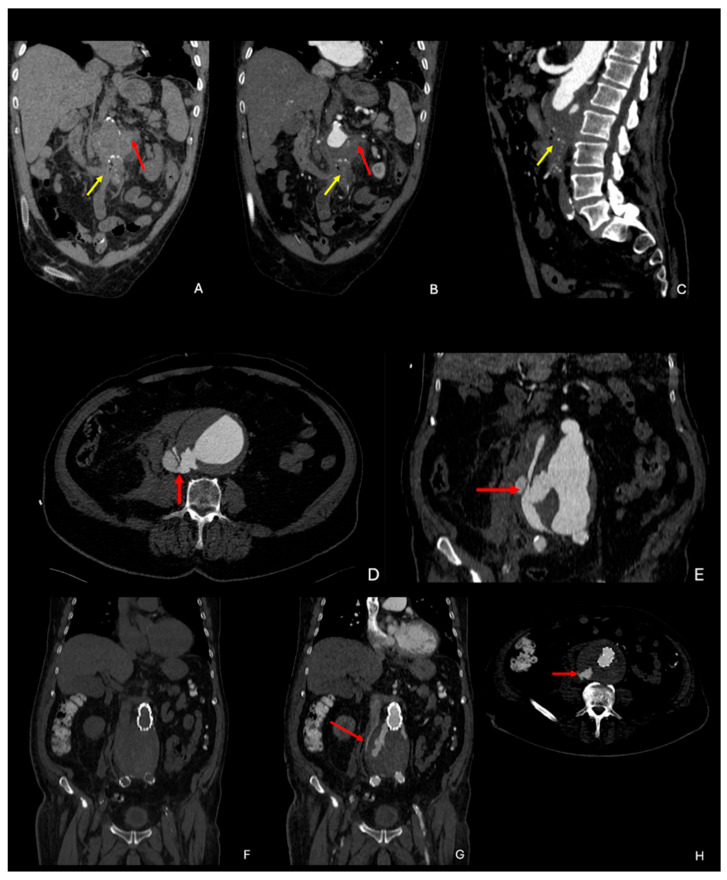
Primary complications of AAAs. (A) Coronal unenhanced CTA showing retroperitoneal hematoma (red arrow) with air bubbles due to primary aorto-enteric fistula (yellow arrow). (B) Coronal enhanced CTA showing retroperitoneal hematoma (red arrow) and air bubbles due to aorto-enteric fistula (yellow arrow). (C) Sagittal enhanced CTA showing retroperitoneal hematoma with air bubbles (yellow arrow) due to aorto-enteric fistula. (D,E) Axial and coronal enhanced CTA in arterial phase showing an aortocaval fistula (red arrow). (F–H) Coronal unenhanced CTA, and coronal and axial enhanced CTA in arterial phase, showing the same patient as in (D,E) after undergoing EVAR, with type 2 endoleak from the IMA and lumbar arteries, and the persistence, although smaller, of the aortocaval fistula (red arrow). CTA: computed tomography angiography; AAA: abdominal aortic aneurysm, IMA: inferior mesenteric artery.
